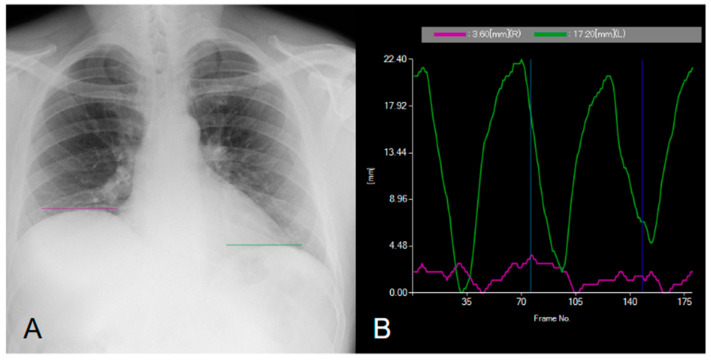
Dynamic digital radiography confirming right hemidiaphragm paralysis. In (A), a dynamic acquisition of the chest in the posteroanterior projection is shown. In (B), the curves represent diaphragm dynamics: the system automatically tracks the highest point of each diaphragm dome and displays the movement using colored curves. The purple curve represents the right diaphragm, while the green curve represents the left diaphragm [1,2]. The movement of the right diaphragm is significantly reduced, indicating diaphragmatic dysfunction and confirming the clinical suspicion. In contrast, the movement of the left diaphragm is regular (Supplementary Material Video S1). The diagnosis of diaphragm dysfunction can be challenging [3]. Standard chest X-ray may suggest motion impairment or paralysis but fails to predict diaphragm function [3,4,5].
